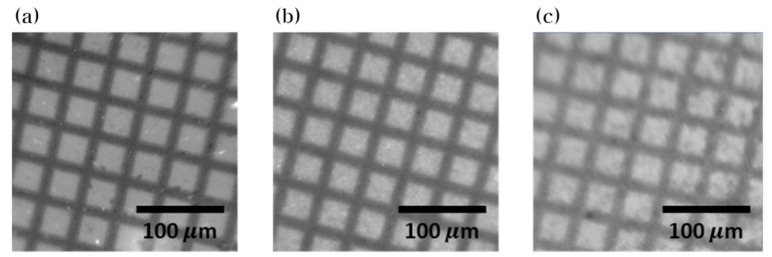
X-ray images of a TEM grid obtained from utilizing ZnWO_4_ scintillator screens with average particles sizes of (a) 176.4 nm, (b) 626.7 nm, and (c) 2.127 μm.
